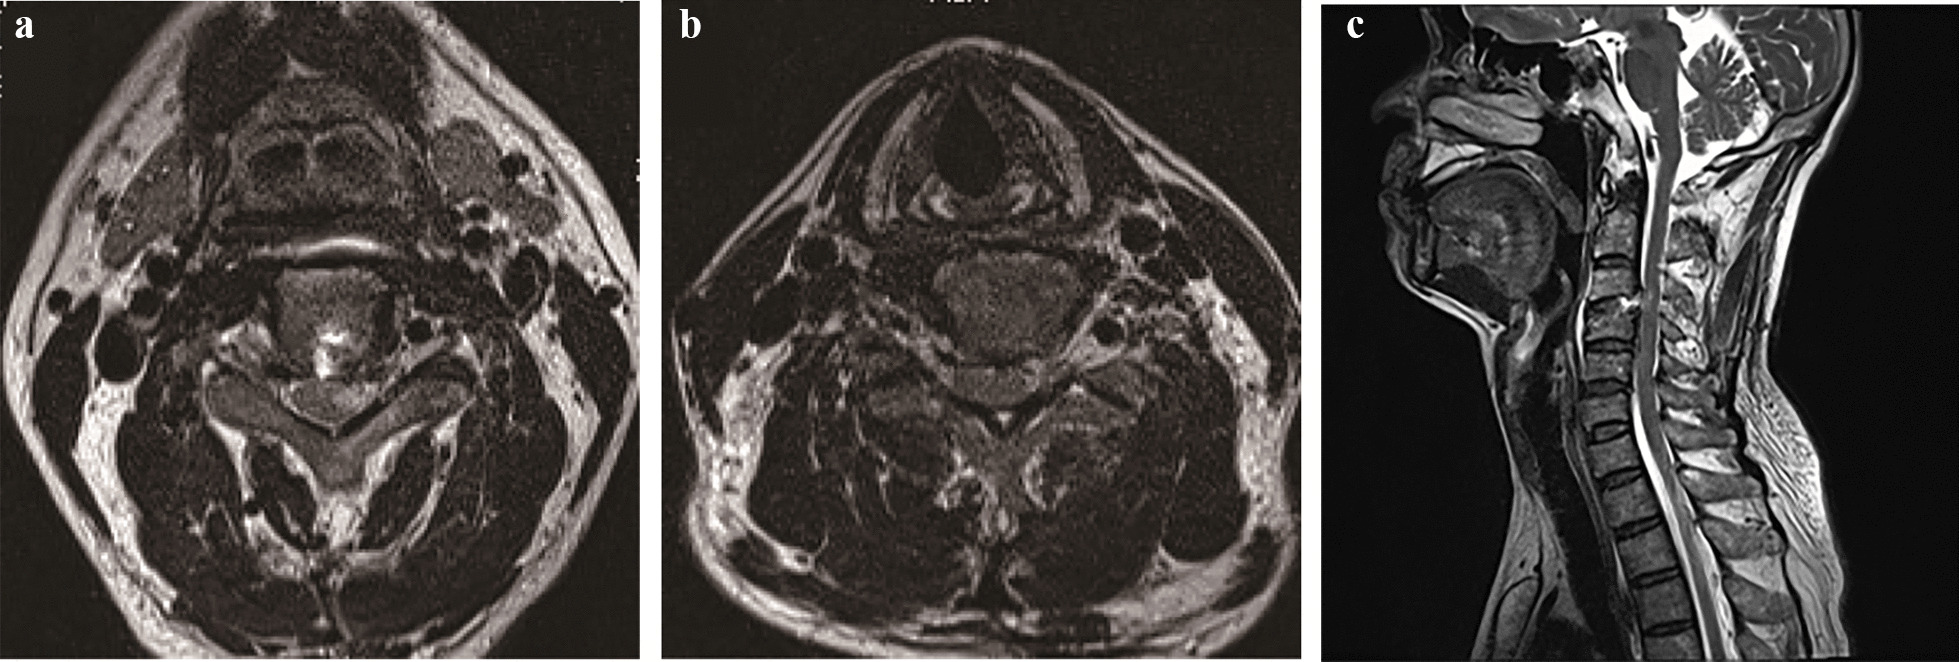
MRI images of the patient 1 week after surgery. a, b T1-weighted axial view shows that the discs compressing the spinal cord at the C3/4 and C4/5 levels were extracted. c T2-weighted sagittal view shows that the patient’s spinal canal is patent and the spinal cord compression has been released
